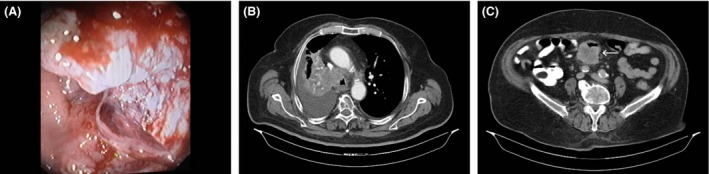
(A) Endoscopic view of the descending colon showing an ulcerated and bleeding lesion. (B) Chest computed tomography demonstrating the adenocarcinoma in the right upper pulmonary lobe. (C) Abdominal computed tomography showing an intraluminal mass in the descending colon (white arrow).
